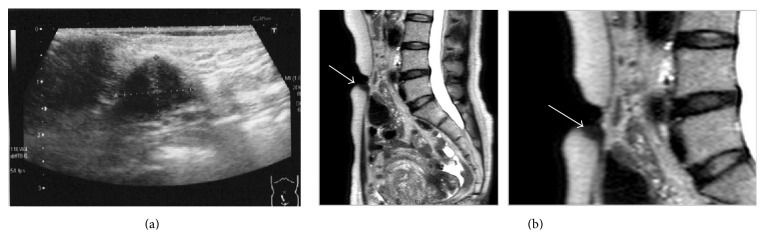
(a) Transcutaneous USG and (b) abdominal MRI (T2-weighted sequence). USG showed 1.0 × 1.5 cm umbilical nodule. MRI also exhibited the hypodense umbilical nodule (arrow) and myoma (asterisk).
